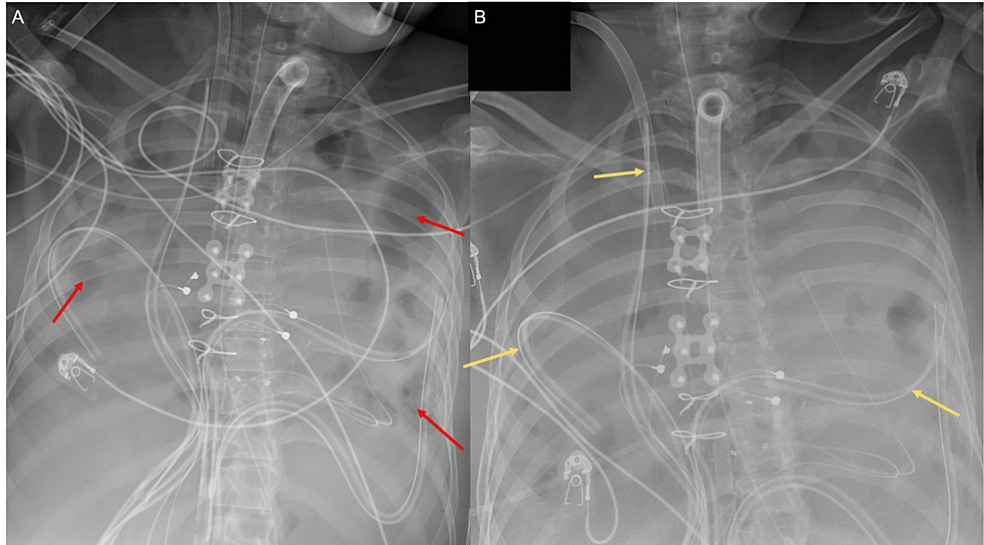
Portable semi-erect anterioposterior radiographs of the chest (A, B) demonstrate bilateral large pleural effusions with areas of loculations. Focal areas of aeration are present (red arrows in A). The patient is status post-sternotomy with sternal wires and closure devices in place. Numerous thoracic drains are in place (yellow arrows in B).
